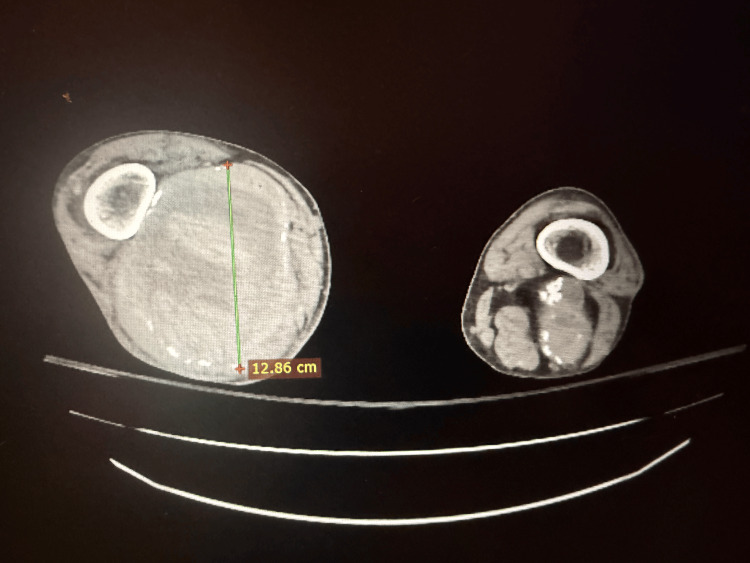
CT angiography revealing an enormous PAA PAA: popliteal artery aneurysm
